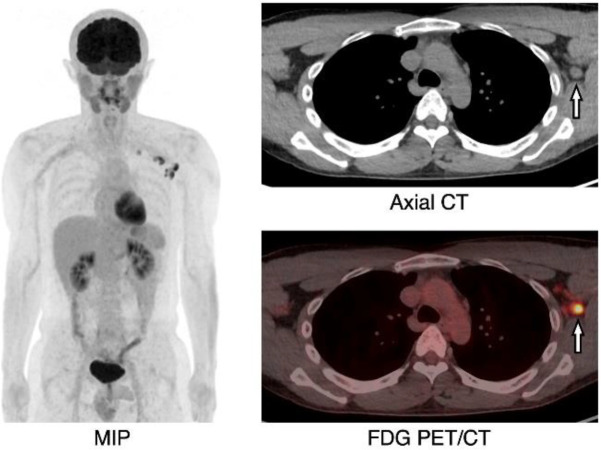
A 40-year-old male, FDG PET/CT (MIP: maximum intensity projection) showed multiple FDG uptakes in six left axillary lymph nodes from levels 1 to 3 (SUV_max_=3.2–9.9). Axial CT and FDG PET/CT show the largest lymph node with the minimum diameter of >10 mm (arrow, short axis: 12 mm, SUV_max_=9.9)
